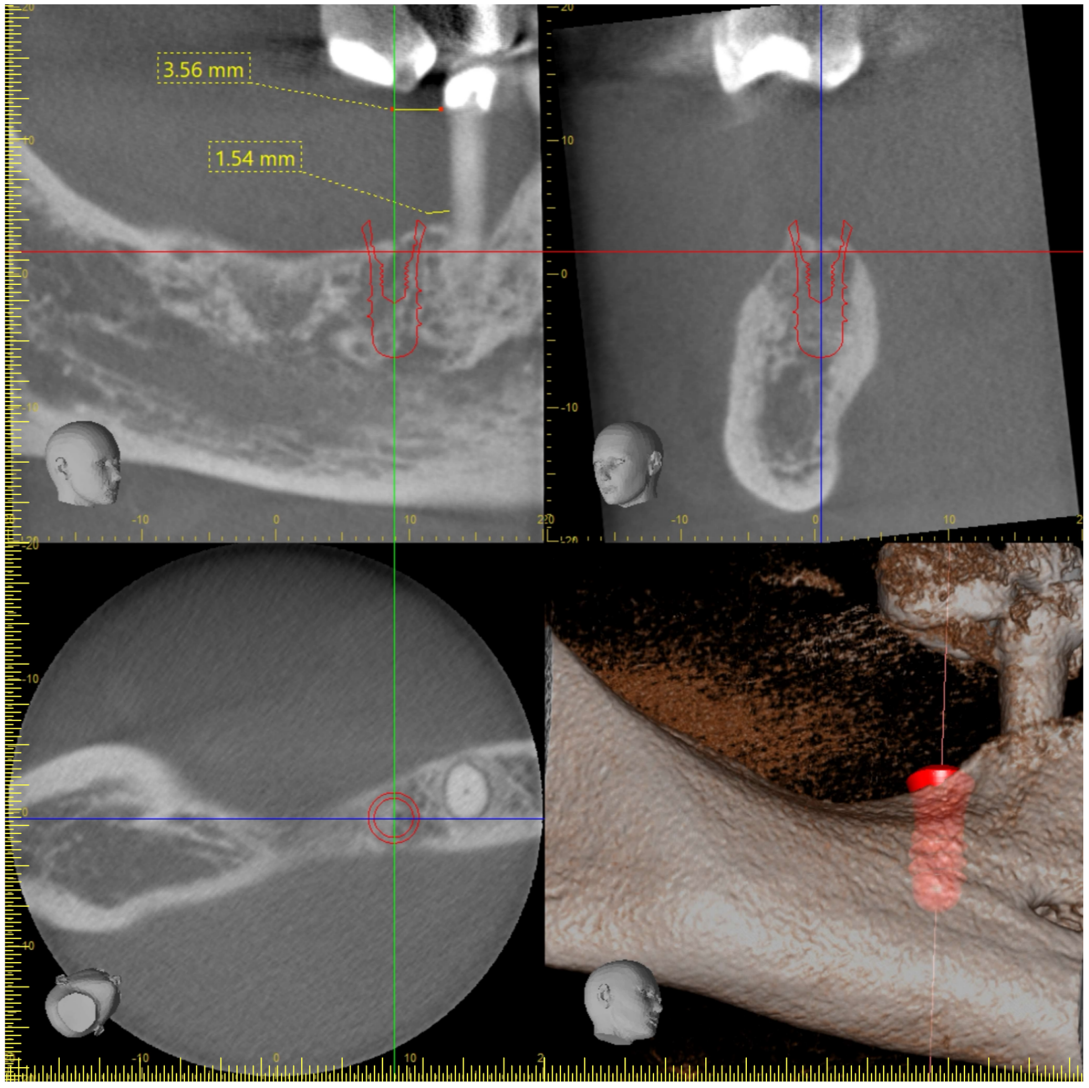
Cone beam computed tomography planning for implant placement in the area of 46
